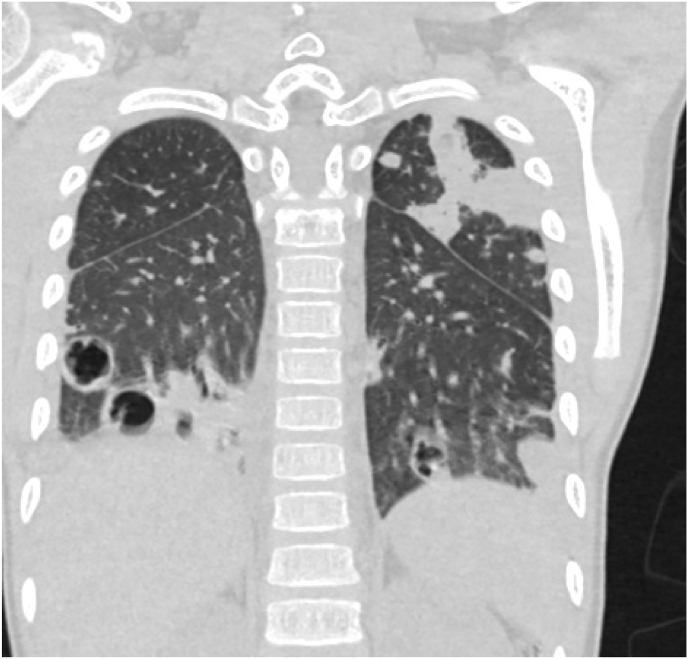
Coronal chest CT (lung window) showing multiple bilateral thick-walled cavitary nodules in both lung lower lobes, septal thickening and thickened pulmonary infiltrates. Left pleural thickening with feeding vessel signs was also noted.
